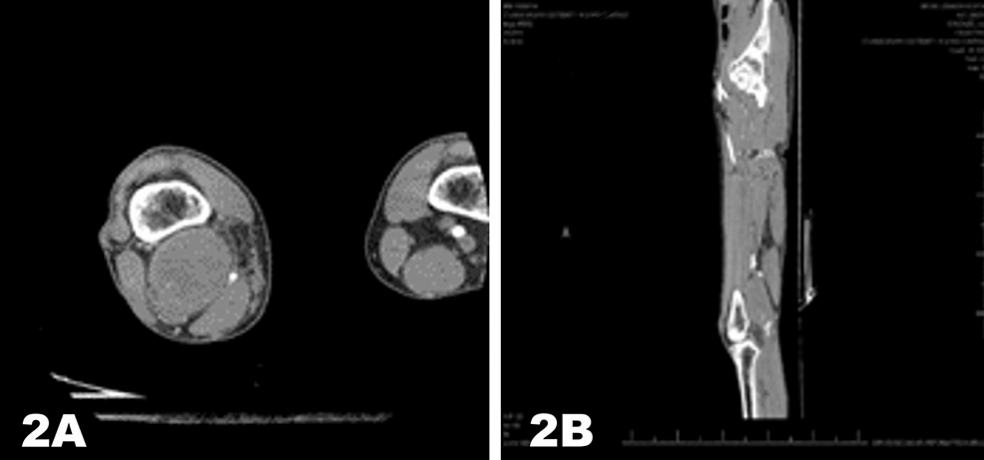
Repeat CT angiogram of lower extremities CT angiogram of the lower extremities demonstrating an enlarging thrombosed popliteal aneurysm in the left popliteal artery (2A) with patent flow through the reverse venous bypass graft (2B).
